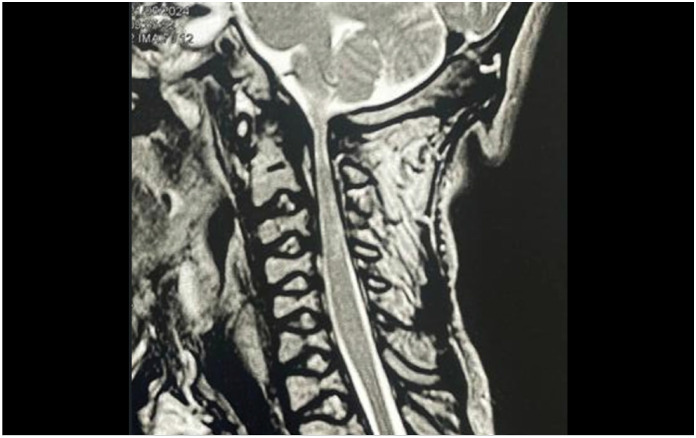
MRI image of the cervical spine showing spinal cord compression and myelopathy.
